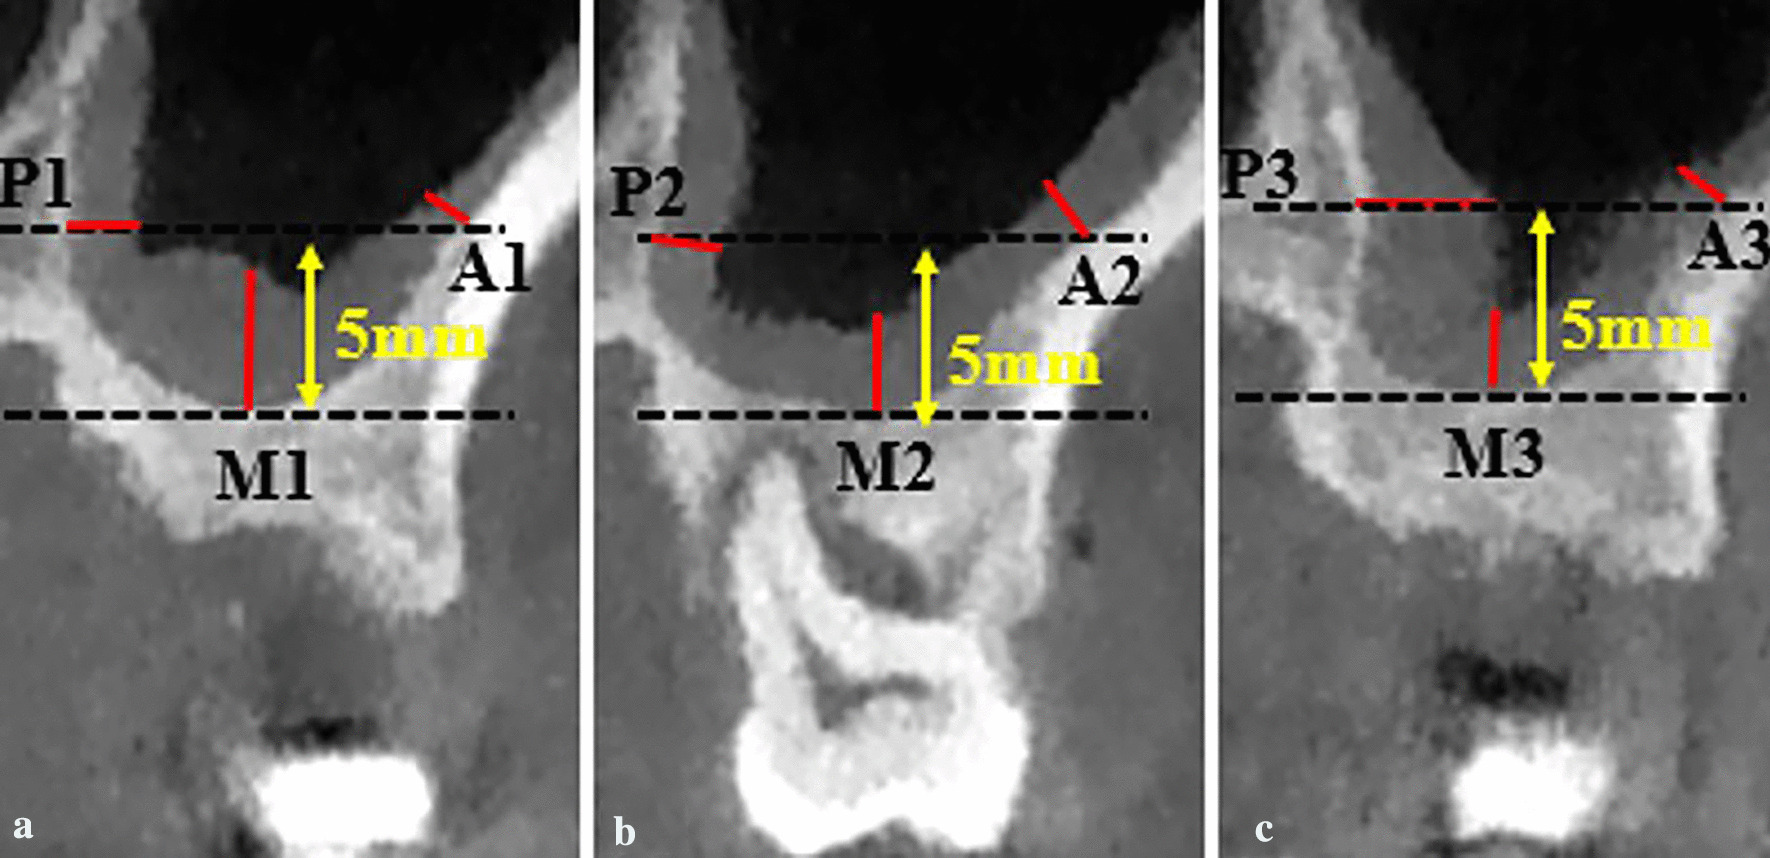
Landmarks for the measurement of MT in coronal CBCT slices. The measurement points of anterior wall and posterior wall of maxillary sinus were all obtained at 5 mm from sinus floor. a the mesial region of pre-extraction CBCT image; b the central region of pre-extraction CBCT image; c the distal region of pre-extraction CBCT image
